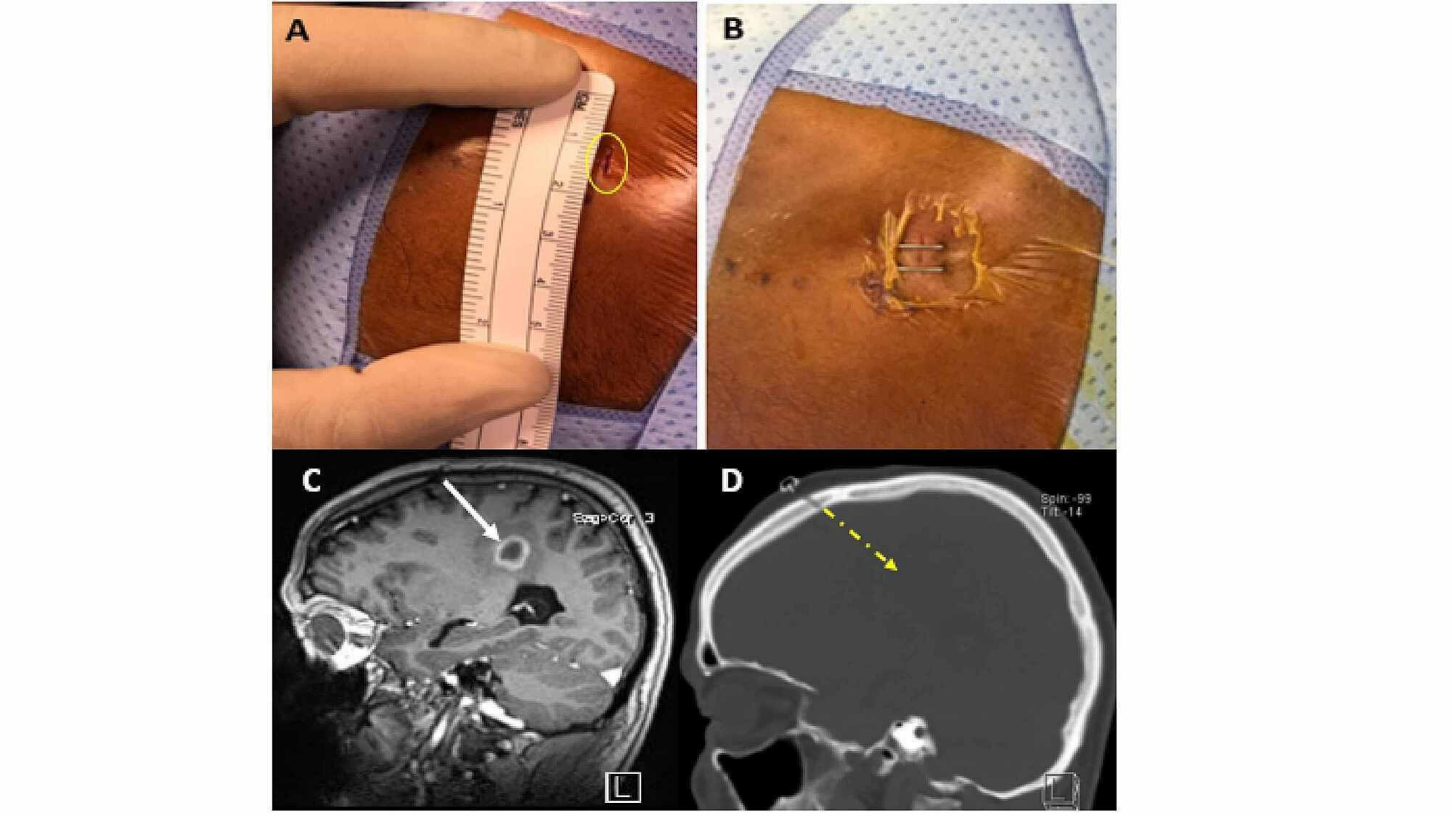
Incision size (A). Closure using two staples (B). Preoperative MRI (T1 with contrast) of a case with enhanced brain lesion in the left fronto-parietal region (white arrow) (C). A 3-mm burr hole as it appears in a postoperative CT head bone window, for the same case, with the trajectory direction (dotted arrow) (D).
